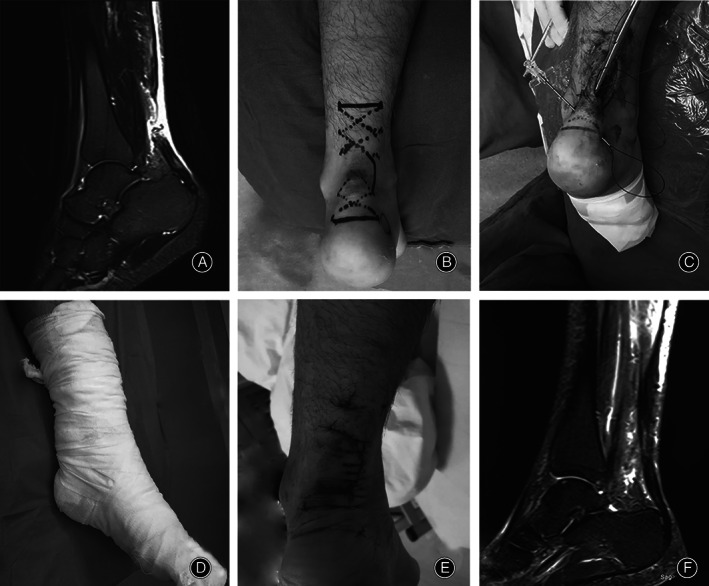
A 29‐year‐old male with a ruptured Achilles tendon because of playing basketball. (A) Preoperative left lower limb MRI (magnetic resonance imaging, Siemens 3.0T) showed rupture of Achilles tendon; (B) before the operation, plan the suture route; (C) during the operation, with the help of epidural puncture needle, the PDS II thread passes through the Achilles tendon; (D) after the operation, the plaster was fixed; (E) the picture of the surgical incision when changing the dressing after the operation; (F) postoperative MRI picture.
